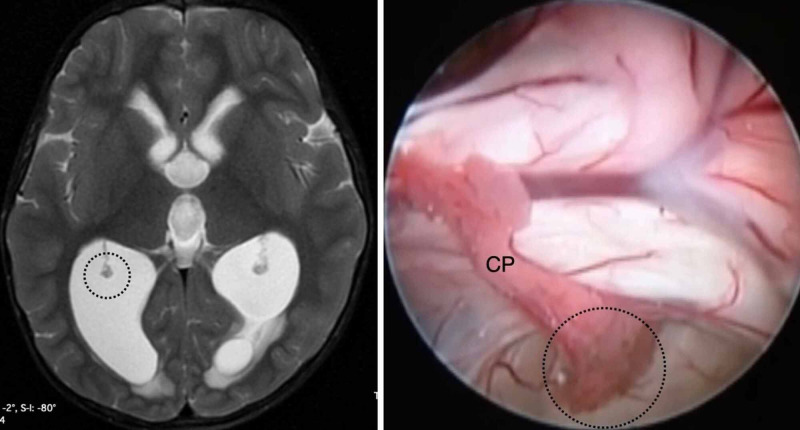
The type I anatomic variation of the glomus of choroid plexus (GCP). Type I GCP is classified as mobile, and the elongation is minimal. On both sides, the GCP (indicated by the black dotted circle) does not reach the middle of distance between the fixation and the posterior limit of the occipital horn, as observed in an axial view on T2-weighted magnetic resonance imaging (left). In this endoscopic view of the right lateral ventricle, the GCP is mobile and not amenable to resection. CP, choroid plexus.
